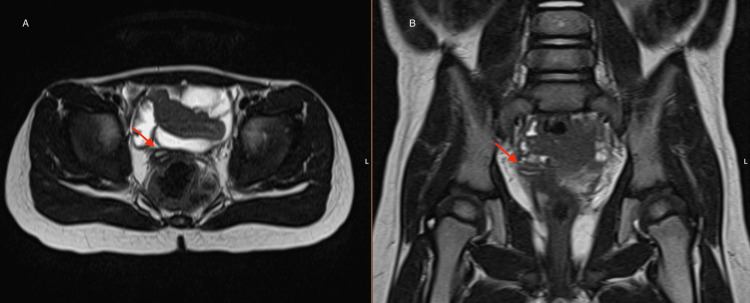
Pelvic MRI confirming OHVIRA syndrome A: Axial plane. B: Coronal plane. MRI: magnetic resonance imaging, OHVIRA: obstructed hemivagina and ipsilateral renal anomaly
